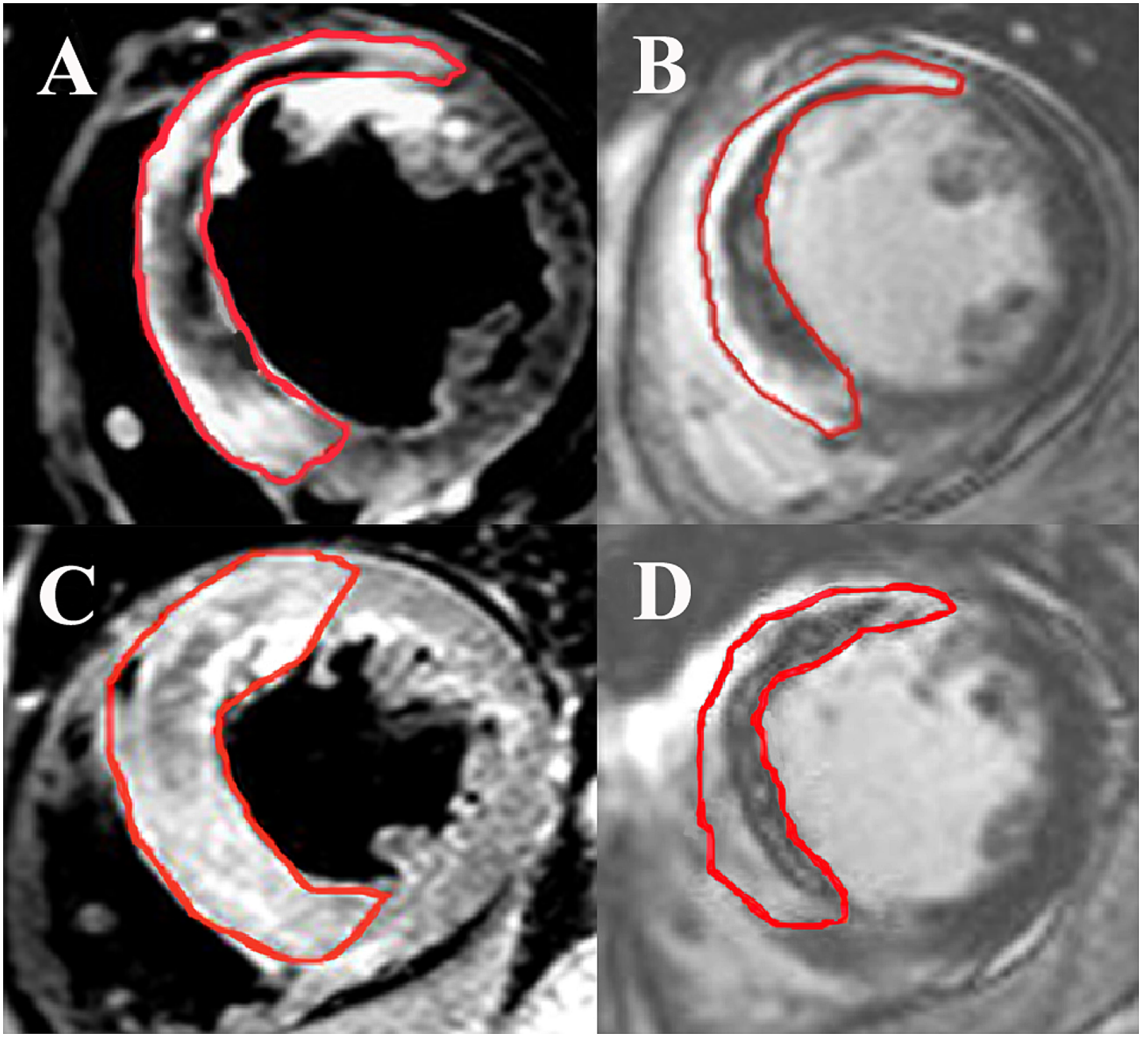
Example Images From a Study of Reperfused Anterior ST-segment Elevation Myocardial Infarction. Representative cardiac magnetic resonance images of a reperfused anterior ST-segment elevation myocardial infarction: (A) high D-dimer level with a short-axis slice of a T2-weighted image, (B) high D-dimer level with a late-gadolinium enhancement image, (C) low D-dimer level with a short-axis slice of a T2-weighted image, (D) low D-dimer level with a late-gadolinium enhancement image. In these cases, D-dimer levels, area at risk, and infarct size were 1.43μg/mL versus 0.26μg/mL, 72.2% versus 55.6% and 38.6% versus 17.9%, respectively, yielding a myocardial salvage index of 46.5 versus 67.8.
